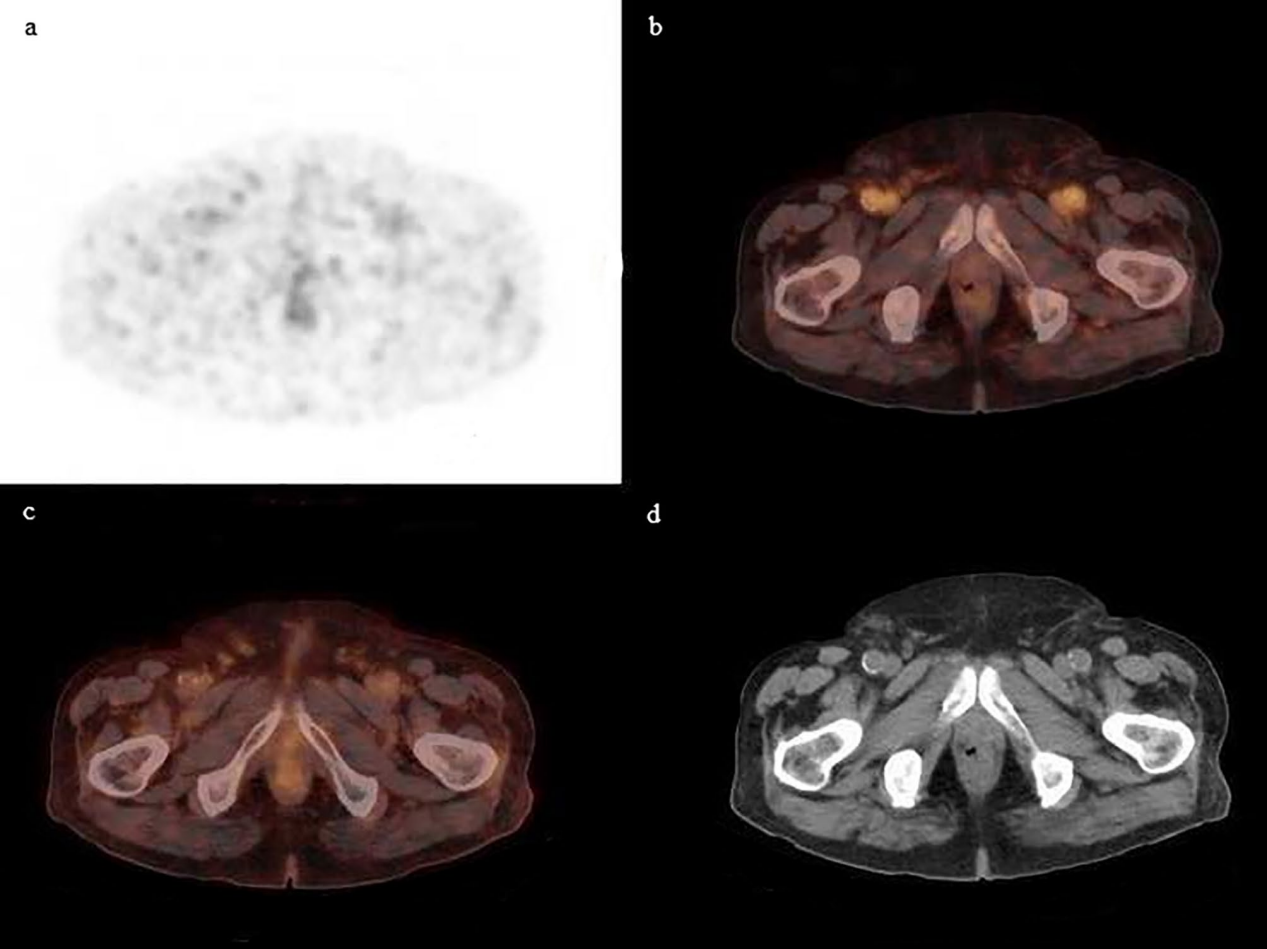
A 80-year-old patient status post radical prostatectomy. PSA level at time of acquisition: 1.38 ng/mL. Whole body ^68^ Ga-PSMAPET/CT was performed: axial cuts of PET (a), fused PET/CT (b, c), and CT images (d) showing no evidence of radiotracer avid disease in the whole body notably in the prostate bed.
